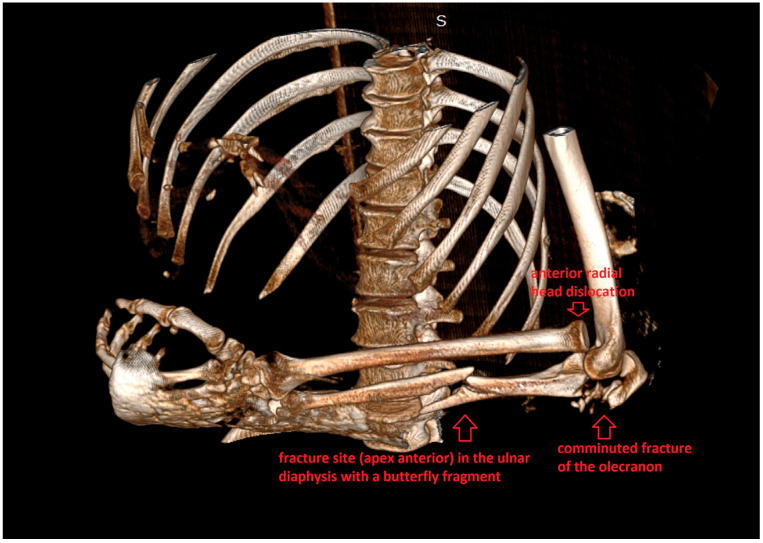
Preoperative 3D CT reconstruction of the Monteggia lesion.
